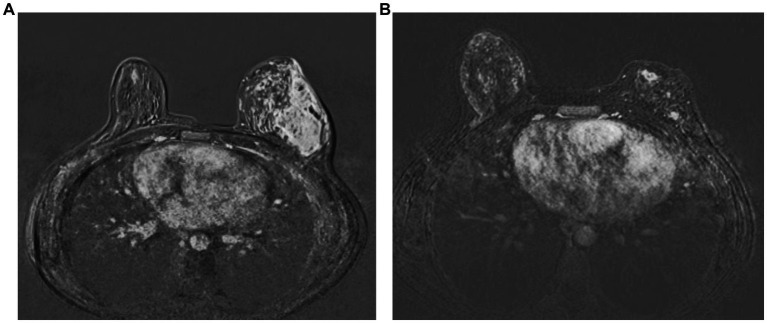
Complete radiological response following completion of ozone therapy as seen in a patient as disappearance of abscess formations and rim-like enhancements and diffuse oedema in contrast-enhanced subtraction images in MRI before (A) and after ozone therapy (B), respectively.
